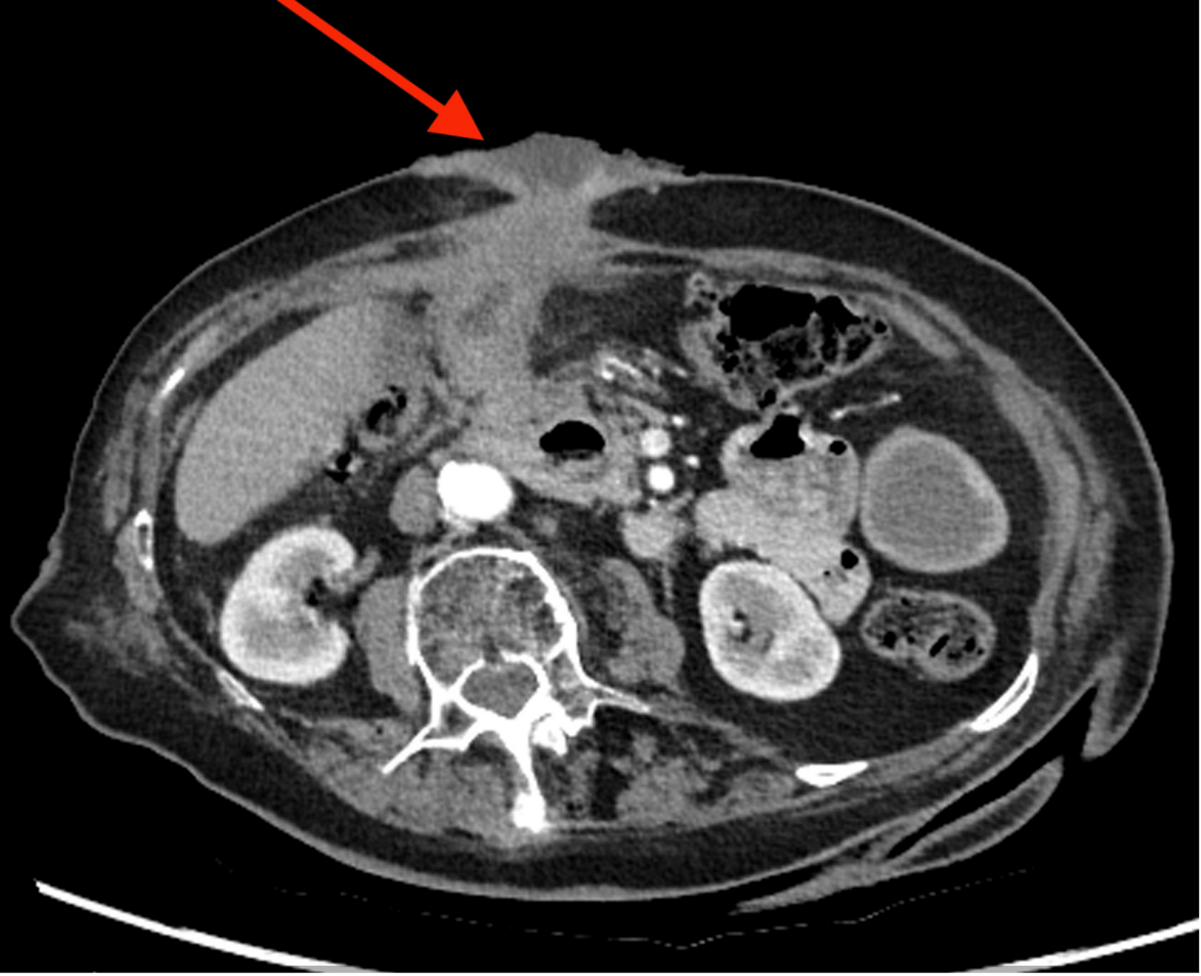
CT abdomen and pelvis with IV contrast (axial) showing a CCF along the drain tract with significant local inflammatory soft tissue thickening (red arrow) CCF: cholecystocutaneous fistula; CT: computed tomography; IV: intravenous
